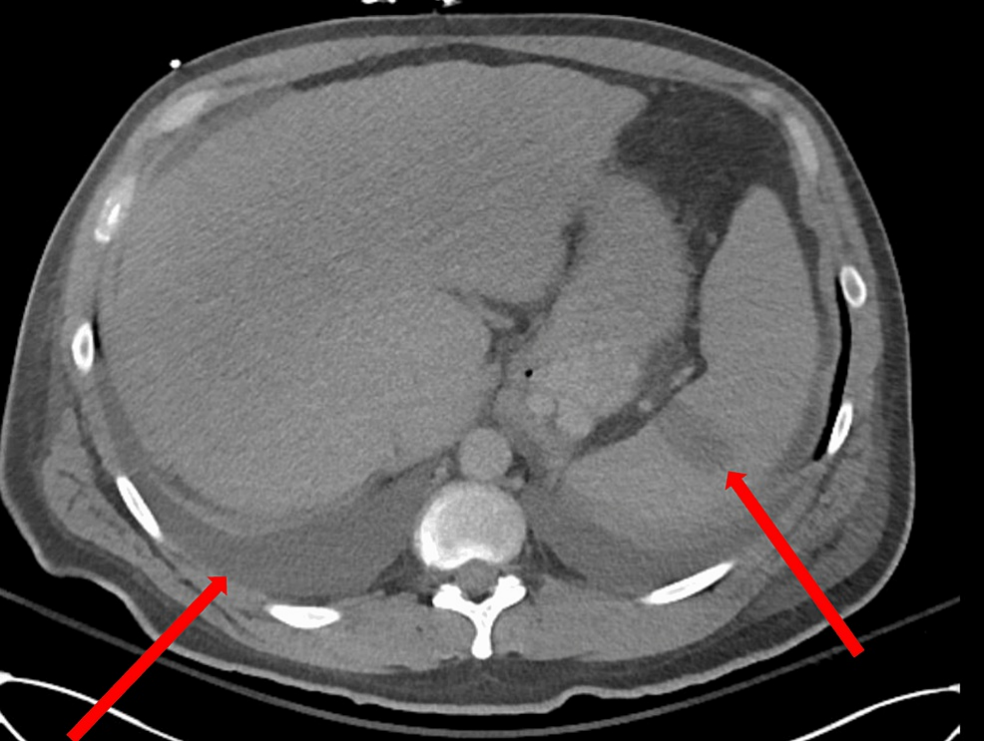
A non-contrast CT scan of the abdomen demonstrates splenic infarct and small pleural effusions (arrows).
